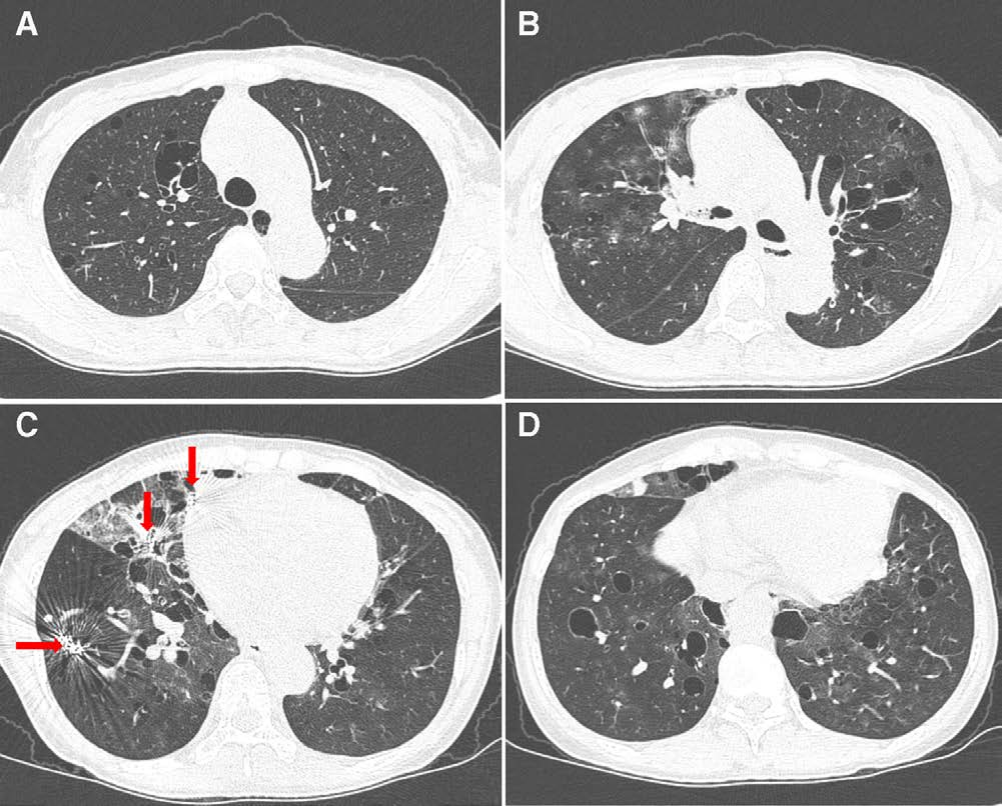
Chest CT performed after 5 years due to hemoptysis. Chest CT images showed multifocal ground glass appearances in both lungs and aggravating state of multiple irregular air-cysts with thin walls since 2010. Previous embolized coils are visible in the right middle lobe and right lower lobe (red arrows). CT = computed tomography.
